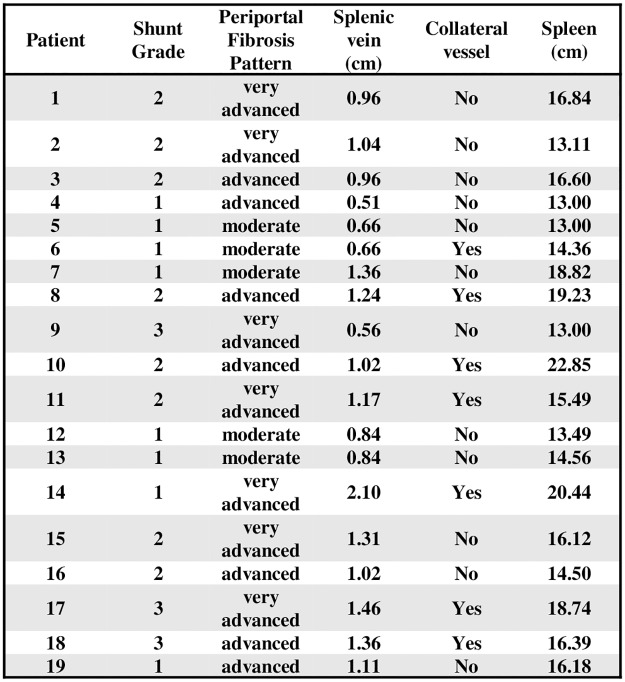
Main abdominal ultrasound findings in the 19 patients with pulmonary shunts.
